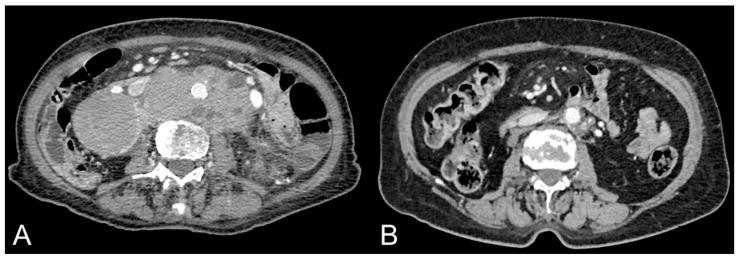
Contrast-enhanced CT images of a 84-year-old female patient with lymphoma showing mass-like abdominal lymphadenopaties (A). Note the complete response after treatment, with resolution of the mass together with increase in VAT and SAT (B).
